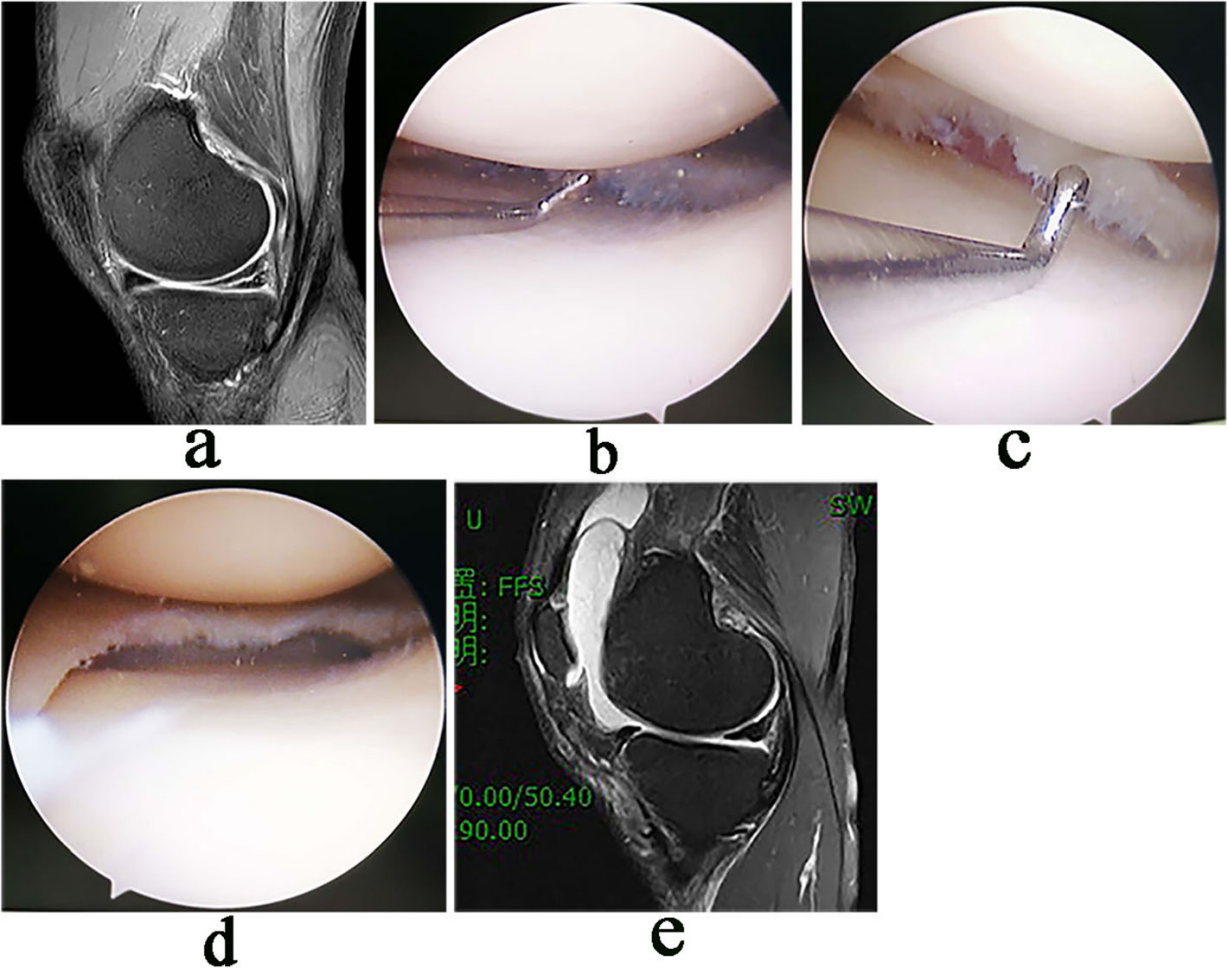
Arthroscopic pie-crusting release of PMC for meniscoplasty. a Preoperative MRI showed a horizontal tear of the medial meniscus. b Arthroscopic visual field of the posteromedial space was not clear, and the probe tip could not pass vertically through the narrow gap. c After release, the probe tip could pass vertically through the narrow gap, and the visual field was improved. d Meniscoplasty of the posterior meniscus was performed without iatrogenic cartilage injury. e After 18 months follow-up, the shape of the posterior meniscus was satisfactory
